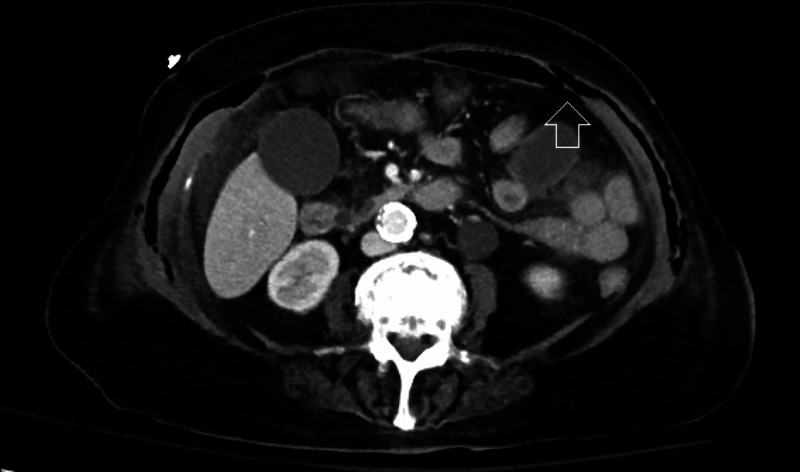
CT scan – axial view – demonstration of subcutaneous air; extensive gas is seen within multiple layers of the abdominal fascia, with the deepest affected layer immediate superficial to the parietal peritoneum (white arrow)
